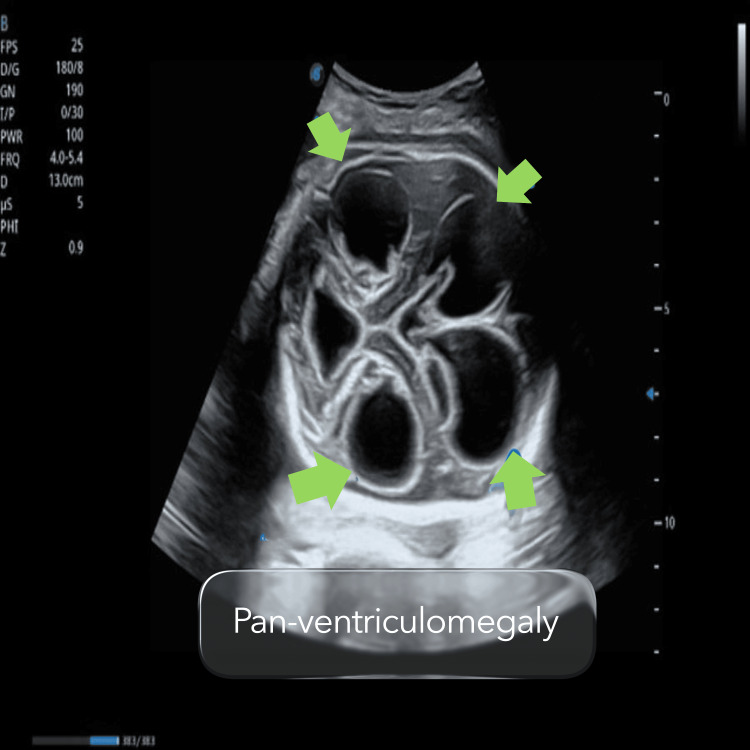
Pan-ventriculomegaly shown in follow-up ultrasound at 32 weeks
